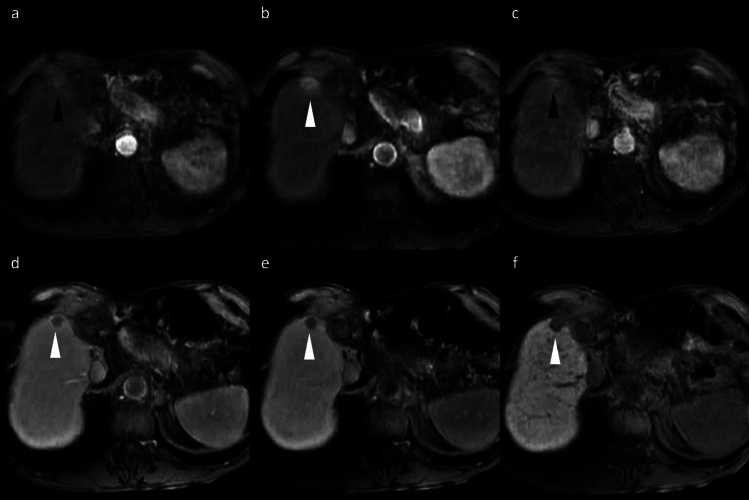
Hepatocellular carcinoma (HCC) in an 80-year-old man with etiology-unknown cirrhosis. Sequential triple arterial phase images are acquired within a single breath hold using gadoxetic acid-enhanced MRI (a–c). The arterial phase images are compromised by transient severe motion; however, the second arterial phase image captures non-rim arterial hyperenhancement of HCC (b; arrowhead). Portal venous washout is seen (d; arrowhead). HCC appears hypoenhancing relative to the background liver in the transitional phase (e; arrowhead) and shows clear hypointensity in the hepatobiliary phase (f; arrowhead)
